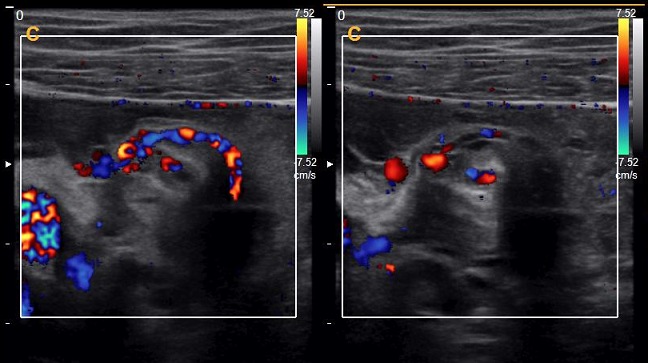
Image doppler de deux tours de spires du pédicule ovarien droit
